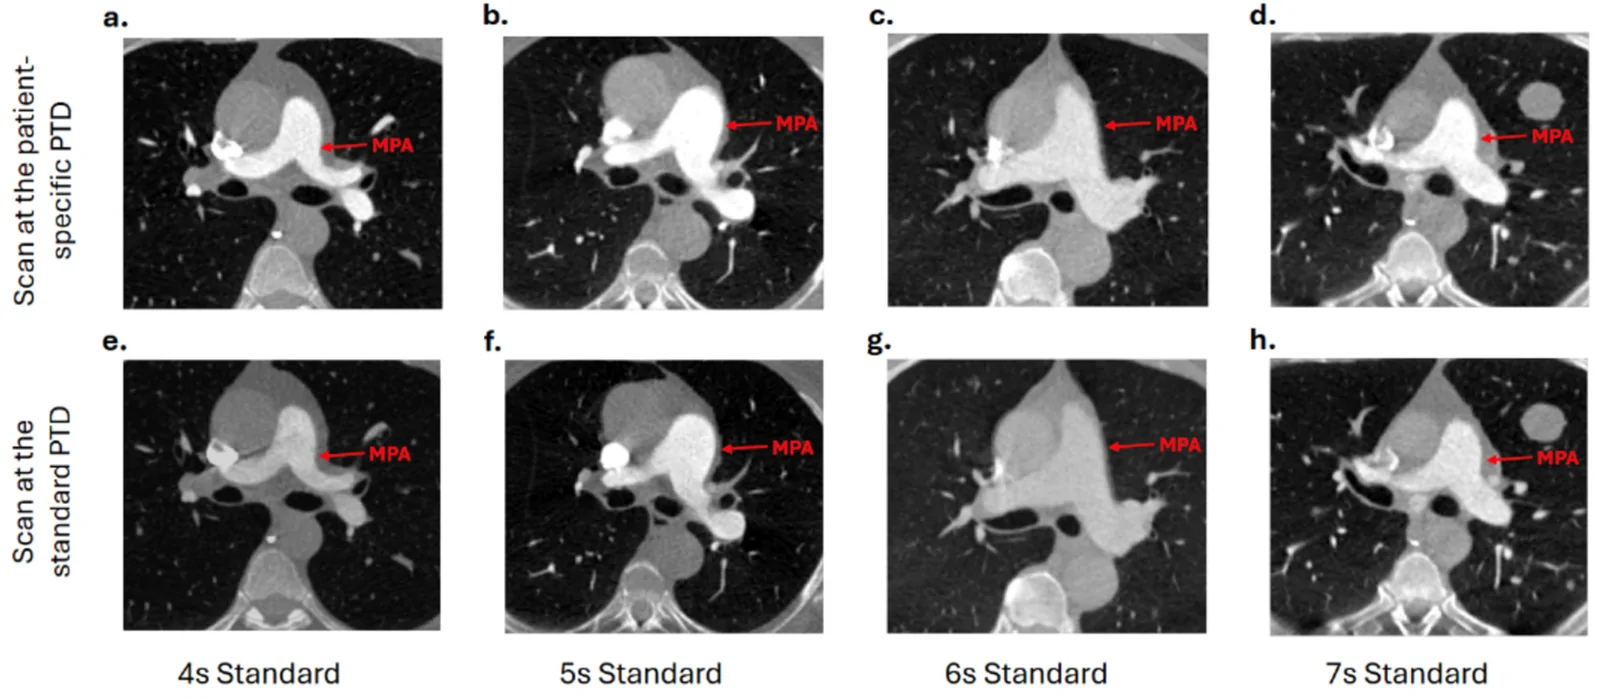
Axial CTPA images at the level of the MPA in four patients (red arrows). The top row (a–d) shows images acquired using the patient-specific PTD. The bottom row (e–h) shows corresponding images from the same patients acquired using fixed-scan delays of 4, 5, 6, and 7 s, respectively. CTPA: computed tomography pulmonary angiography; MPA: main pulmonary artery; PTD: post-trigger delay.
